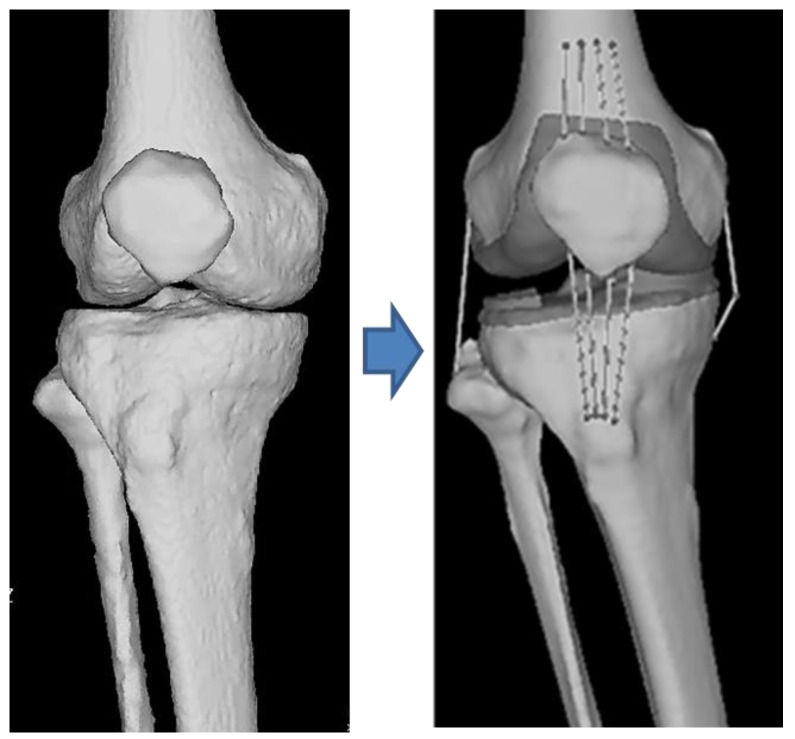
This image is a 3D image obtained by multi-slice CT with ligament, meniscus, and cartilage generated by software.
